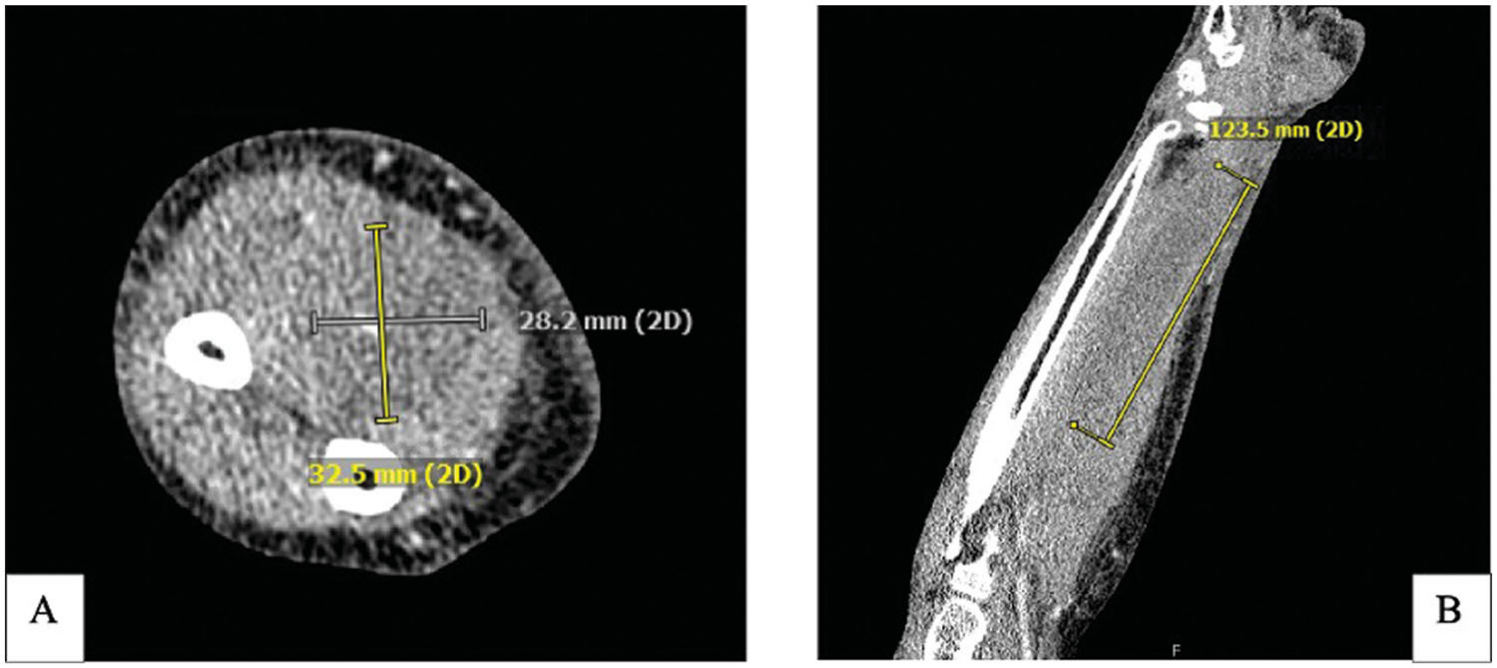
CT Right upper extremity with IV contrast. (A) Axial CT demonstrating a hypodense nonenhancing intramuscular collection within the volar compartment measuring at least 12.3 × 3 × 3 cm. (B) Coronal view of intramuscular collection.
